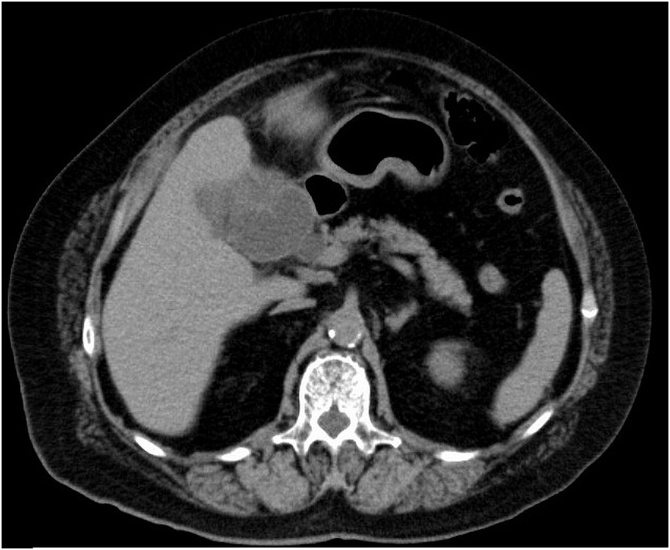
Image from abdominal enhanced computed tomography (CT) before PTGBA. CT revealed an enlarged gallbladder. There was no fluid collection.
